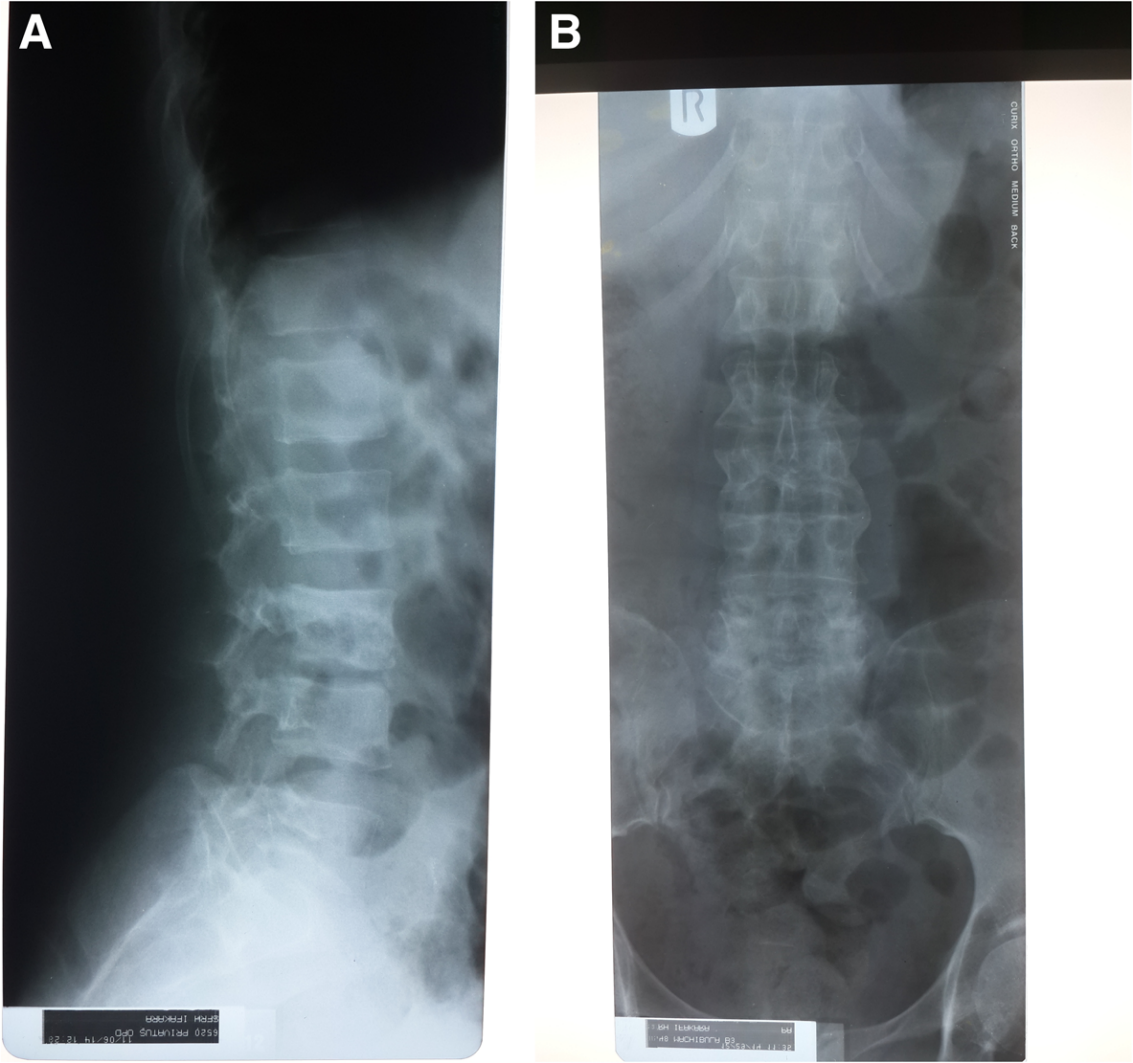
Lumbar Radiographies of Both Patients. a Patient 1: Lumbar lateral X-ray showing harmonic lordosis, regular spinal alignment, normal height of vertebral bodies, noticeable reduction of intervertebral space between L4 and L5, small calcification in the anterior longitudinal ligament between L4 and L5, with no obvious destruction of vertebra L4 and L5. b Patient 2: Lumbosacral X-ray showing straightening of thoraco-lumbar region, regular spinal alignment, reduced height of vertebra L3 with moderate angulation of the vertebral body, lateral and ventral osteophytes, reduced intervertebral spaces, maximum at L3/L4 and sclerosis of intervertebral joints maximum at L4/L5 and L5/S1
